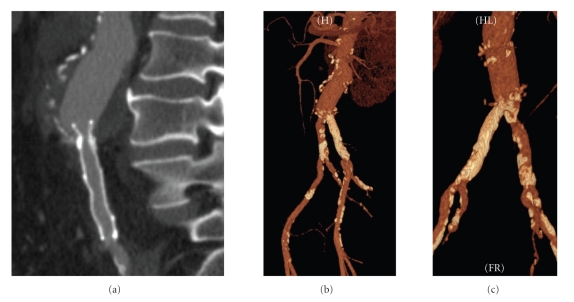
(a) Multidetector computed tomography (MDCT) image (multiplanar reconstruction) showing adequate stent expansion covering the distal graft anastomosis. (b) and (c) Tridimensional reconstruction (MDCT images, lateral and posterior views) showing the heave calcified lesions.
